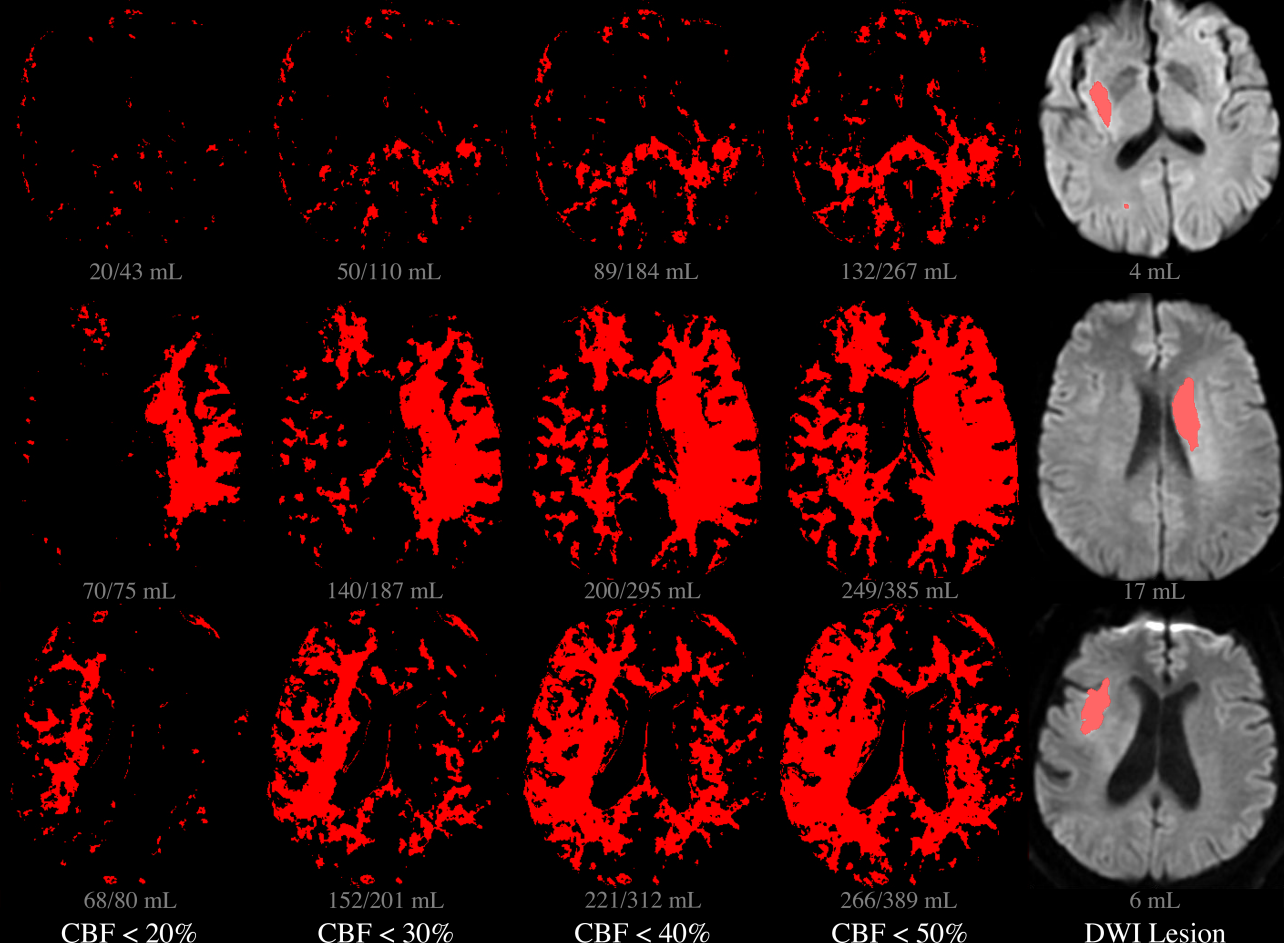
Core lesion volumes derived from various CBF thresholds. Each row depicts a single slice location, in each of three different patients. In the leftmost four columns, red pixels are those in which CBF was below each of four different relative thresholds: 20%, 30%, 40%, and 50%, i.e. the putative core lesion defined by each threshold. In the DWI image on the right, the red region reflects the actual DWI-based core lesion, as outlined by a radiologist. Volumes below each image reflect the cumulative lesion volume in all sixteen slices, with the volume calculated using the unilateral method appearing before the slash, and the bilateral method’s volume appearing after the slash.
